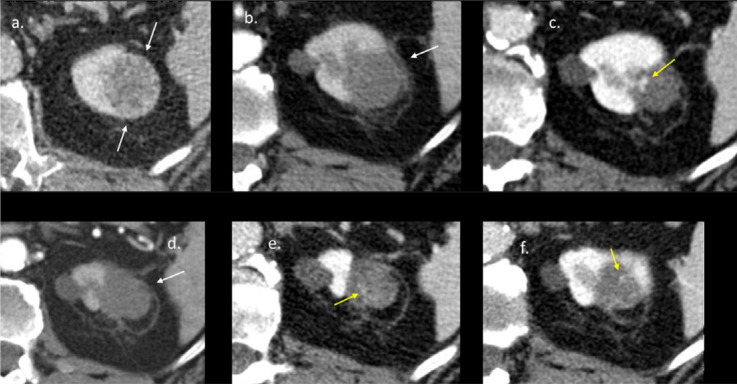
Axial contrast‑enhanced CTs of the patient with two local tumor recurrences. a. RCC lesion in the left lower pole before ablation (white arrows). b. CT scan 3‑month post‑ablation shows no contrast enhancement in the ablation zone (white arrow). c. CT scan 12 months post‑ablation shows contrast enhancement (yellow arrow) in the ablation zone, and thus a local tumor recurrence. d. CT 3 months after the second cryoablation shows no residual tumor in the ablation zone (white arrow). e–f. On the CT 12 (e) and 18 months (f) post‑ablation the contrast enhancement was very subtle (yellow arrow) and stable. Patient is further followed‑up.
